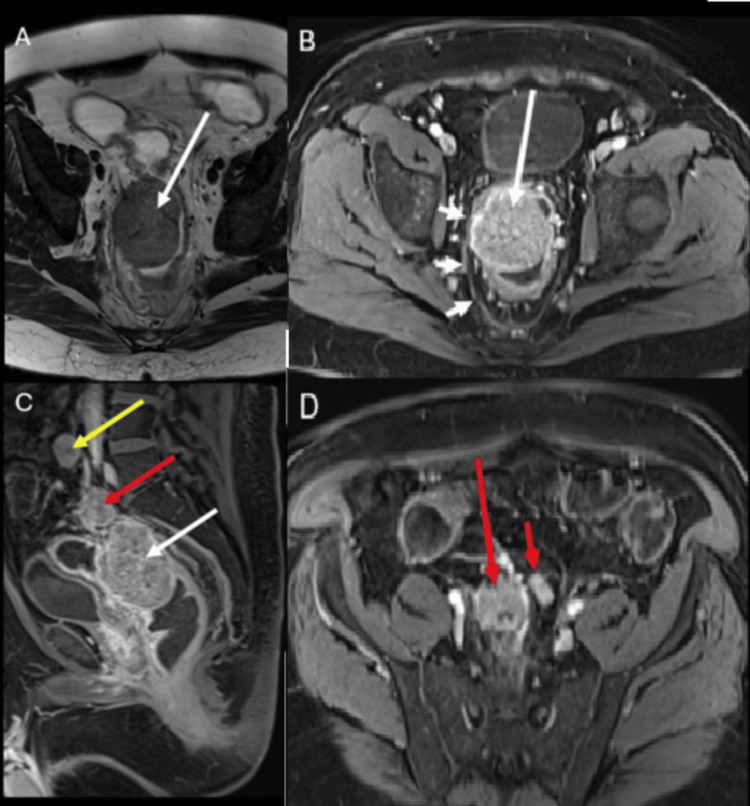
Preoperative MRI scan of the abdomen and pelvis Preoperative axial T2-weighted image (A) and axial contrast-enhanced T1-weighted image (B) of the rectum show the large mass (long white arrows) in the rectosigmoid colon. Note that the mass extends laterally on the right, where it is inseparable from the mesorectal fascia (short white arrows). A sagittal contrast-enhanced T1-weighted image (C) demonstrates the rectosigmoid mass (white arrow), a high mesorectal nodal mass (red arrow) just superior to it, and a low retroperitoneal (extramesorectal) nodal mass (yellow arrow) even more superior. An axial contrast-enhanced T1-weighted image obtained through the upper pelvis (D) shows the high mesorectal nodal mass (long red arrow) seen in figure (C) and a smaller enlarged lymph node (short red arrow) just to the left of it MRI: magnetic resonance imaging
